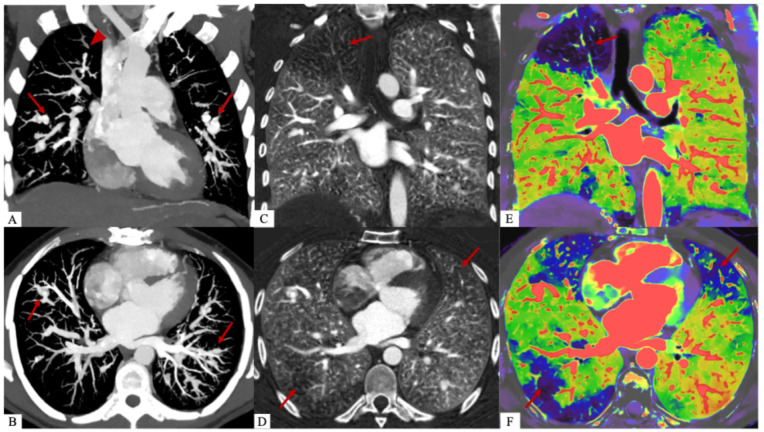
A dual-energy CT scan (Philips CT 7500, Philips Healthcare, Best, The Netherlands) was performed, showing that the pulmonary perfusion defects were consistent with areas of pulmonary embolism. It is also useful for distinguishing the different components, particularly the poorly enhanced myxomatous component from the purely vascular part of pulmonary arterial aneurysms. We calculated the extracellular volume (ECV) of myxomatous aneurysms at 43%, compared to 29% for healthy myocardium. This clearly shows an increase in the extracellular space due to the presence of the myxomatous matrix [1]. Dual-energy CT images with coronal (A) and axial (B) reconstructions at 40 keV and MIP after injection of contrast agent at the pulmonary arterial and aortic phase show bilateral pulmonary arterial vascular aneurysms (*red arrows*) and more hypodense intravascular myxomatous proliferations (*red arrowhead*). Coronal (C) and axial (D) lung iodine non-water maps show perfusion defects in territories of myxoma emboli (*red arrow*). Overlay coronal (E) and axial (F) images of the conventional and iodine density maps representing the same perfusion defects (*red arrows*).
